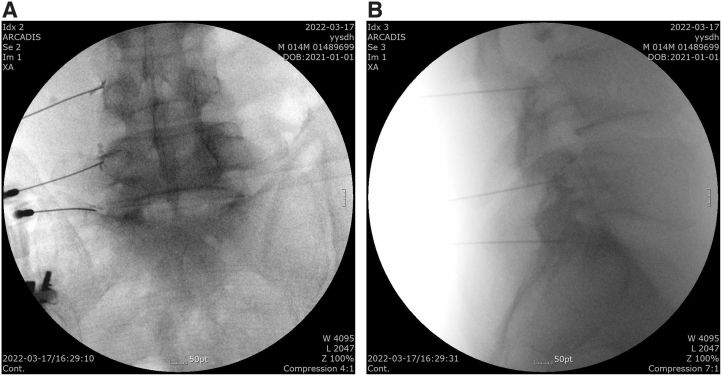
Anteroposterior (A) and lateral (B) radiography showing appropriate needle positioning with contrast dye during medial branch radiofrequency procedure.
